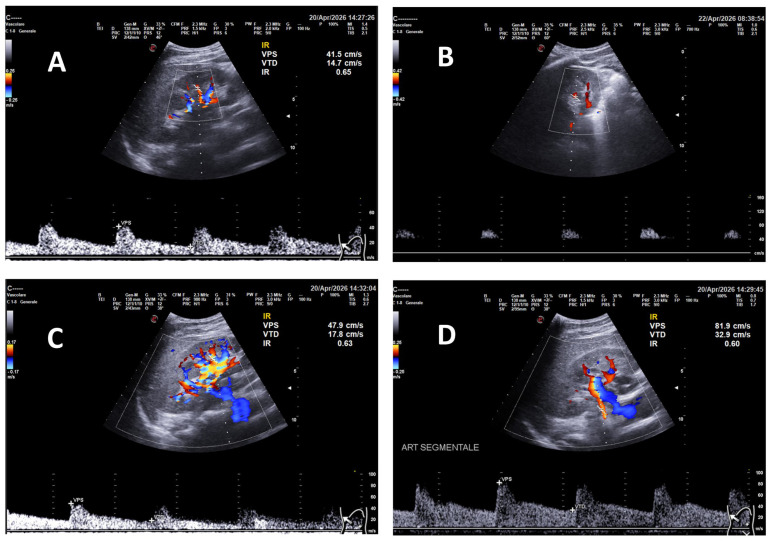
Spectral Doppler waveform analysis and quality control. Device-generated labels are shown as acquired in Italian language. (A) Valid interlobar arterial waveform with labeled peak systolic velocity (“VPS” in figure), end-diastolic velocity (“VTD” in figure), and calculated RRI (“IR” in figure). (B) Invalid trace due to excessive gain obscuring end-diastolic flow. (C) Aliased waveform from inappropriate pulse repetition frequency. (D) Off-target trace from inadvertent segmental artery (“art segmentale” in figure) sampling.
